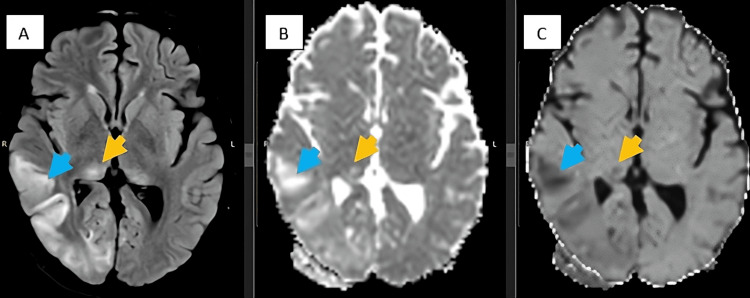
Three-month follow-up brain MRI A: Axial FLAIR shows a right temporo-occipital cortical hyperintense lesion (blue arrow) and bilateral pulvinar nucleus (yellow arrow). B, C: injuries restrict diffusion. FLAIR, fluid-attenuated inversion recovery.
